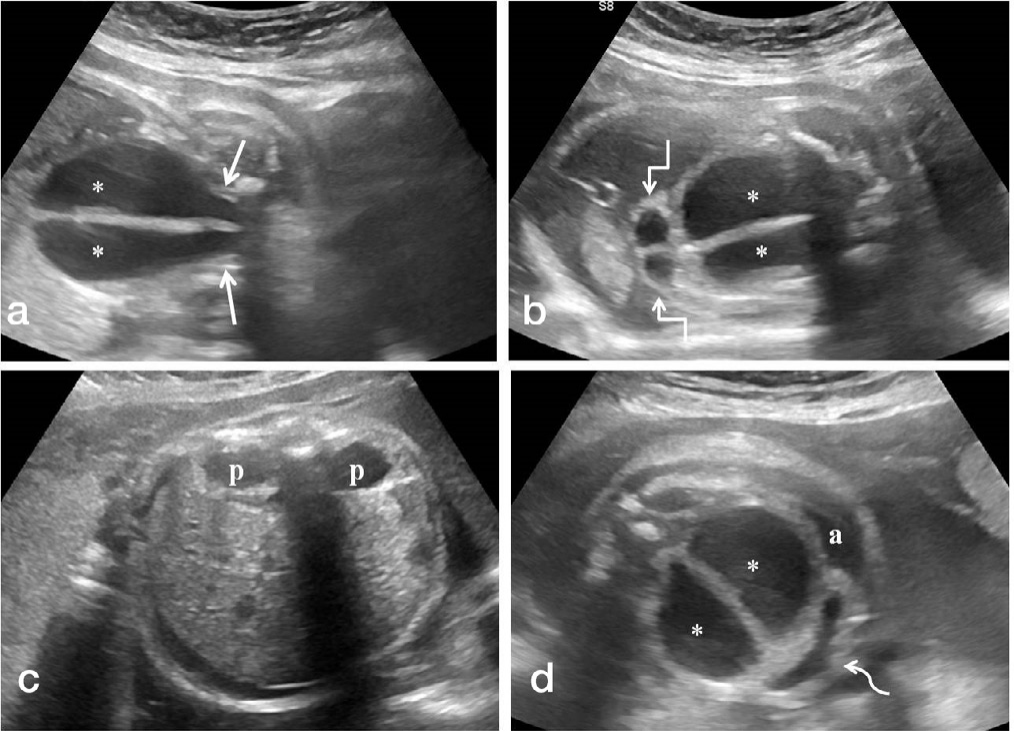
(a-d). Antenatal ultrasound images in coronal plane (a,b) showing the distended vagina (*) with central echogenic vertical septum funneling towards the pelvis (straight arrows) and distended hemiuteri at the cranial aspect of distended vagina (square arrows). Images in the axial plane (c,d) reveal bilateral renal pelvic dilatation (p) and anteriorly displaced urinary bladder (curved arrow) with fetal ascites (a).
